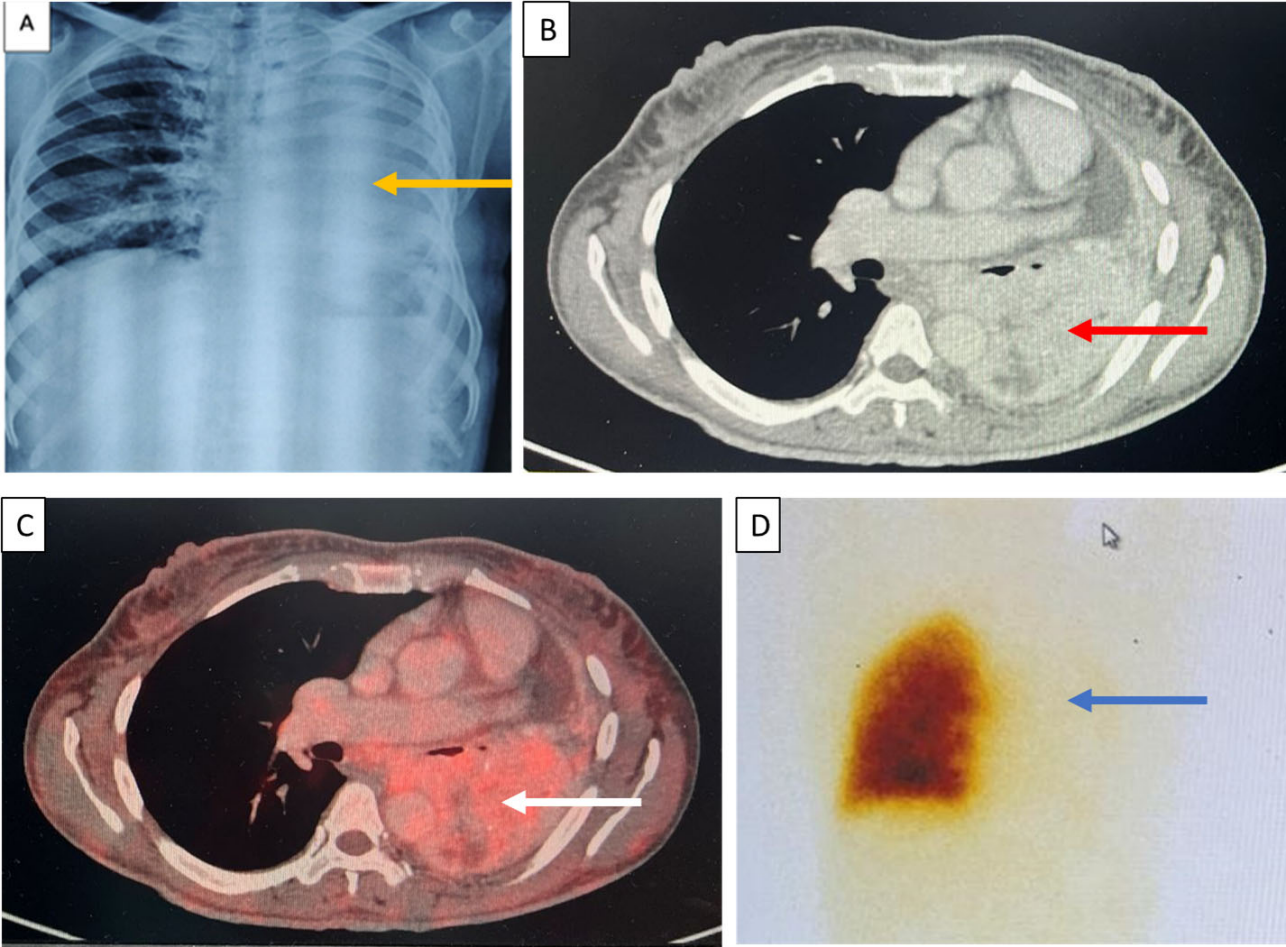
(A) Chest X-ray on day 4 of admission with complete opacification of the left hemithorax (yellow arrows). (B) Contrast-enhanced CT chest showing a heterogeneous mass with intense enhancement in the left lung (red arrow). (C) FDG-PET CT revealing an avid lung mass in the left lung (white arrow). (D) A ventilation perfusion scan suggestive of a severe decreased to negligible perfusion in the left lung (blue arrow).
